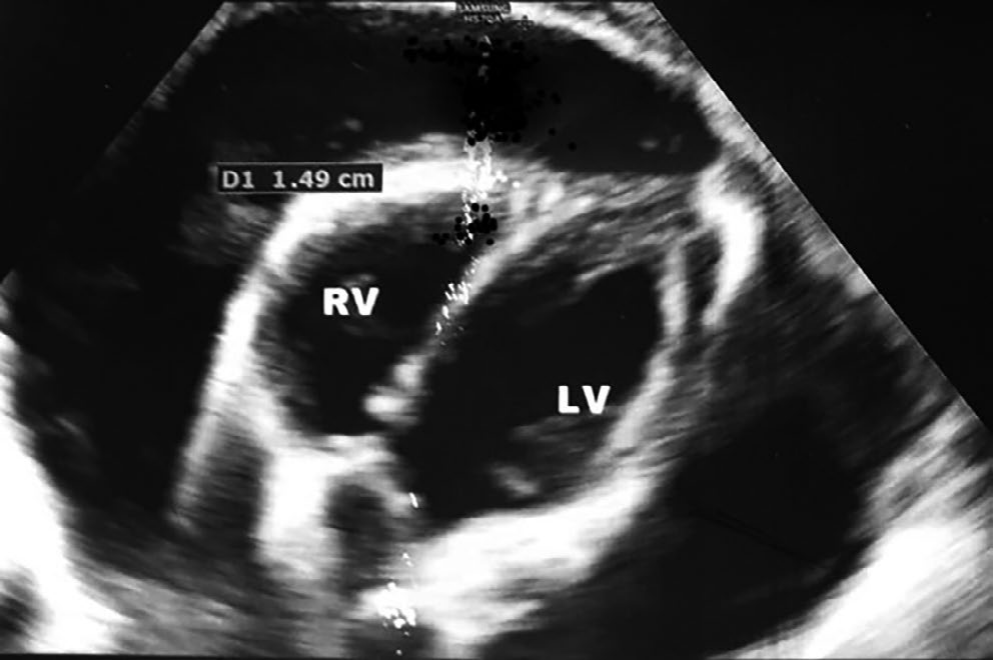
Transthoracic echocardiogram. The heart is seen surrounded by a very large pericardial effusion, without any sign of tamponade sign (15 mm left side & 18 mm right side)
